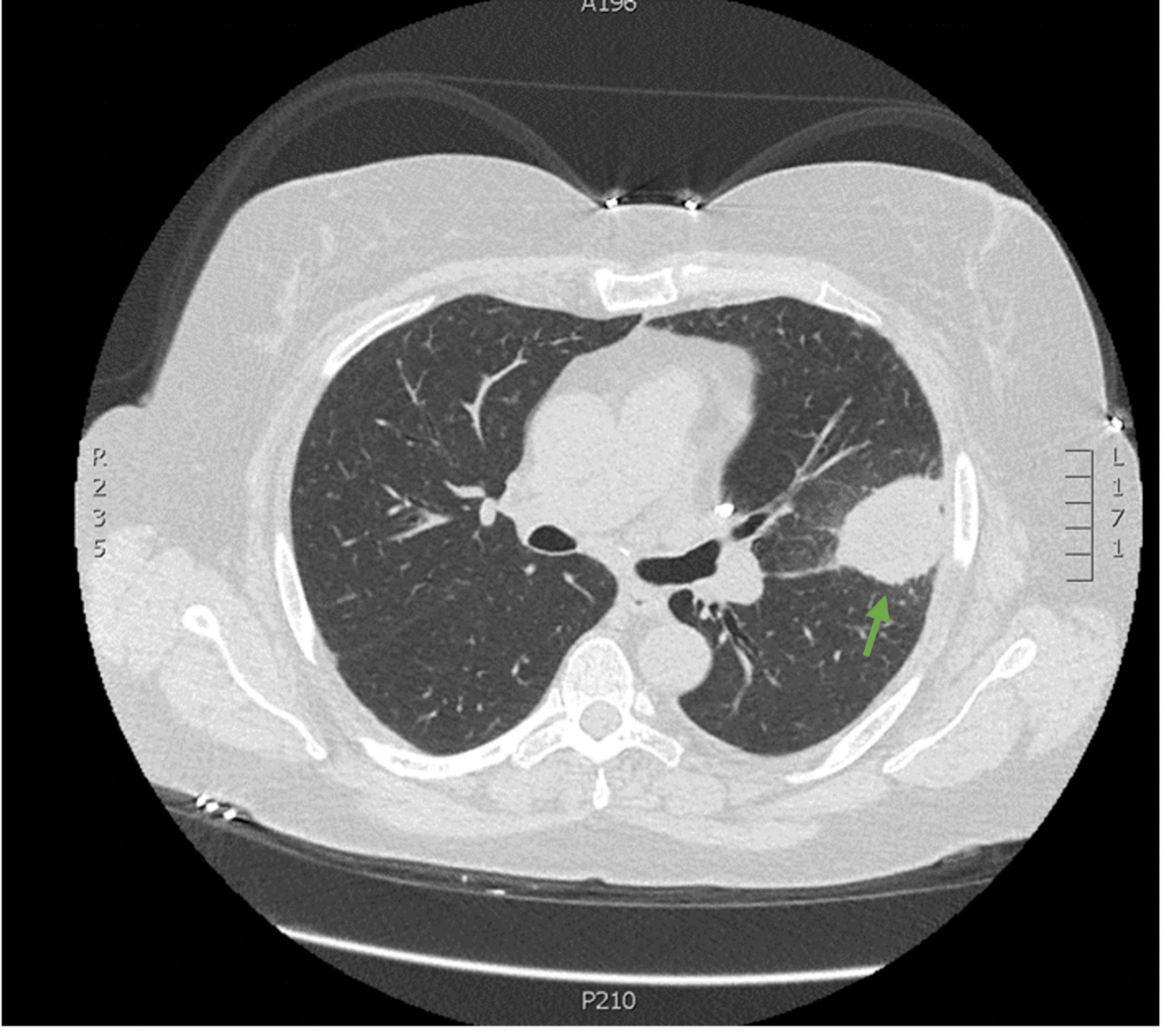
Axial view of the CT chest without contrast revealing a 4x3.5x3.5 cm mass invading the major fissure (green arrow) Slice thickness: 1 mm. Edge-enhanced reconstruction algorithm.
